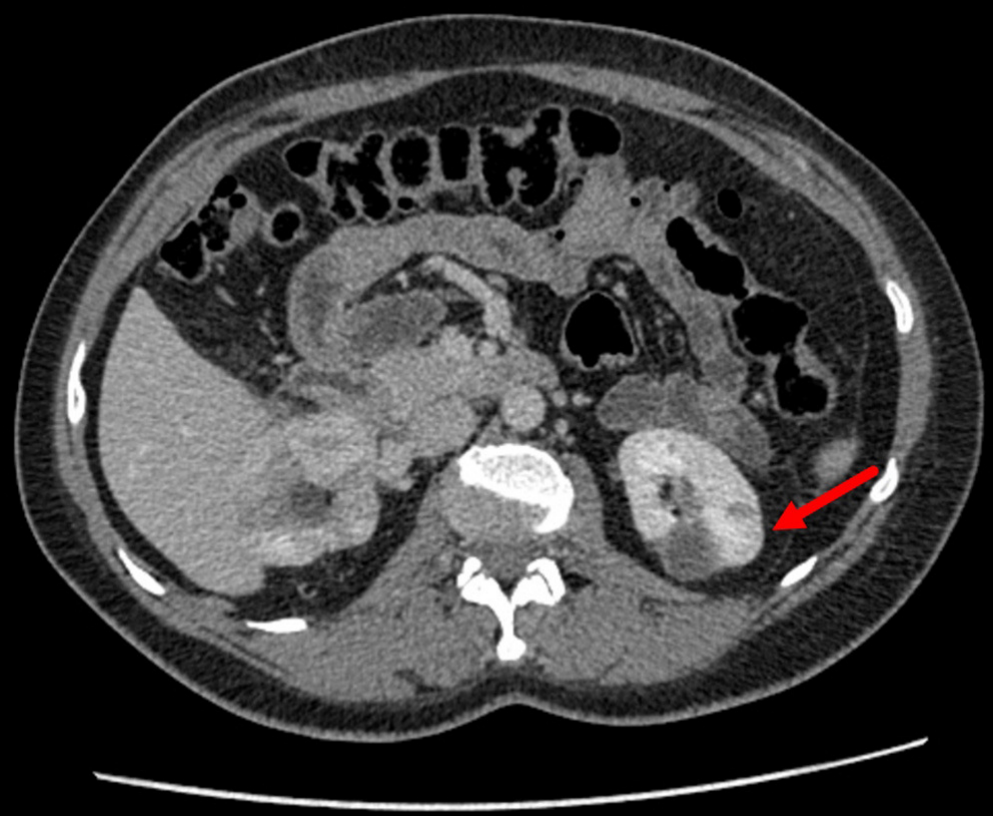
Contrast-enhanced CT of the kidneys in patient K. The left kidney examined prior to the second surgery is indicated.
